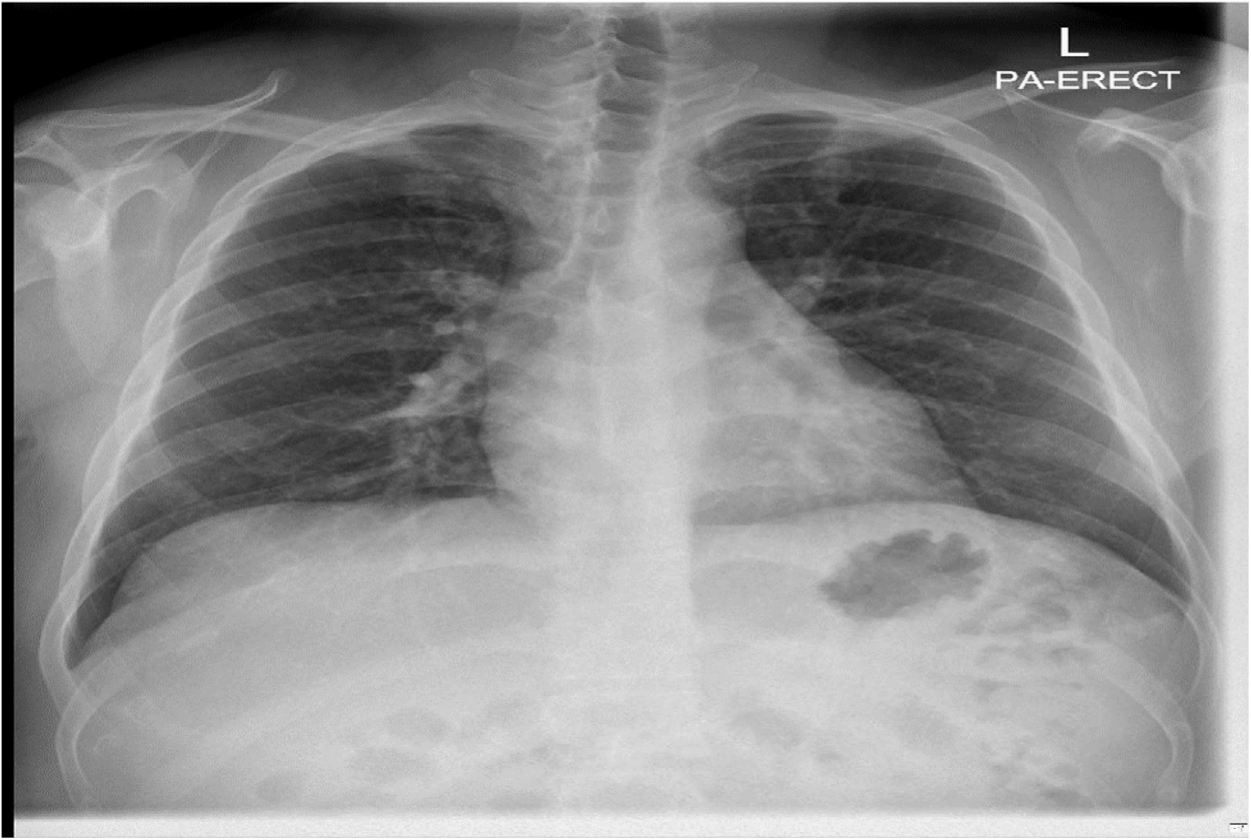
Chest X-ray upon first admission. Cardio-mediastinal silhouette is within normal limits with the heart being normal in size. No pleural effusion / pneumothorax/consolidative patches identified.
